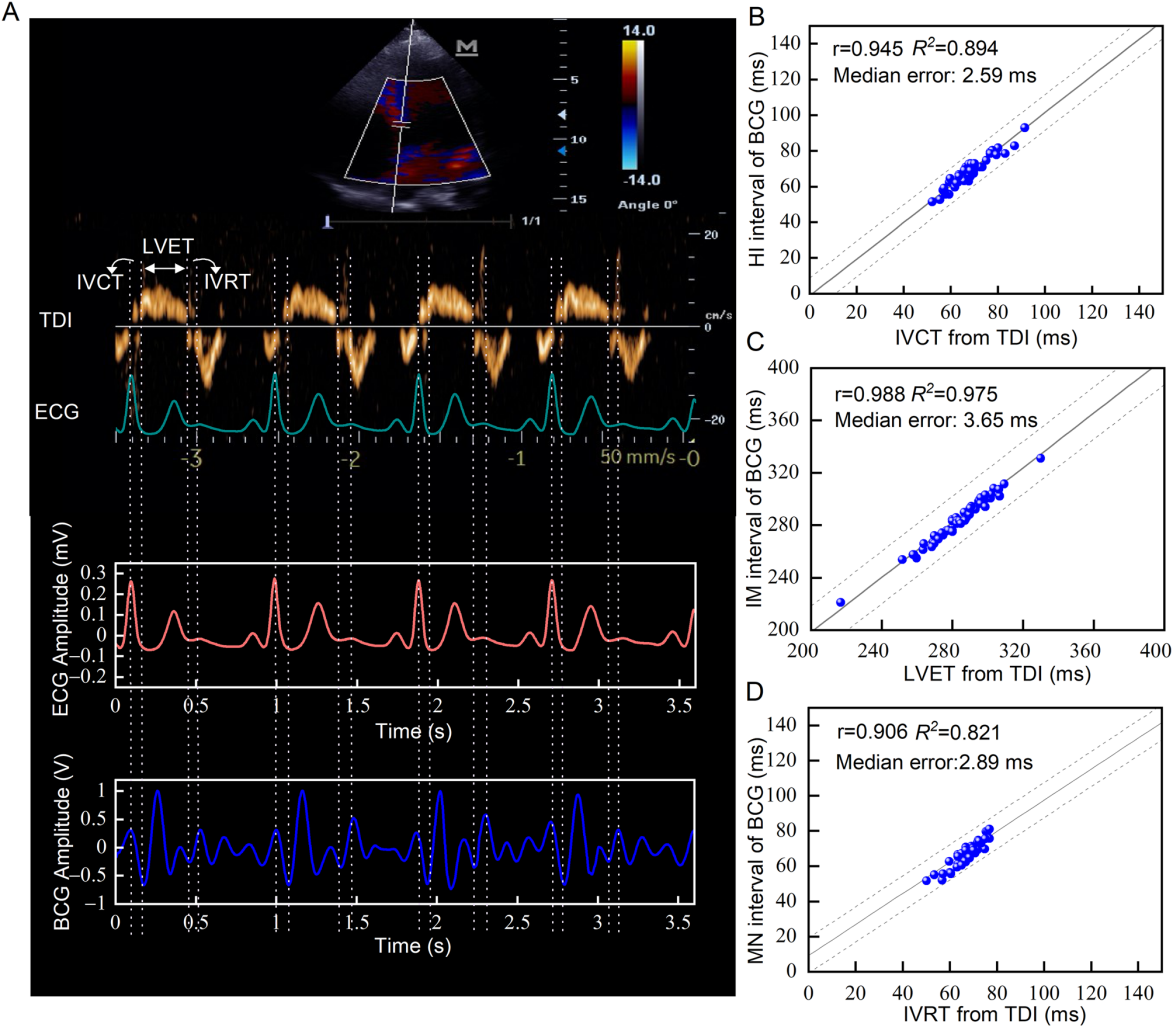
Time parameters measured by BCG and TDI. (A), The IVCT, LVET, and IVRT measured by TDI and correspondingly obtained from the BCG recordings. (B), Correlation between the HI interval and the IVCT. C, Correlation between the IM interval and the LVET. (D), Correlation between the MN interval and the IVRT. BCG, ballistocardiography; IVCT, isovolumetric contraction time; IVRT, isovolumetric relaxation time; LVET, left ventricular ejection time; TDI, tissue Doppler imaging.
